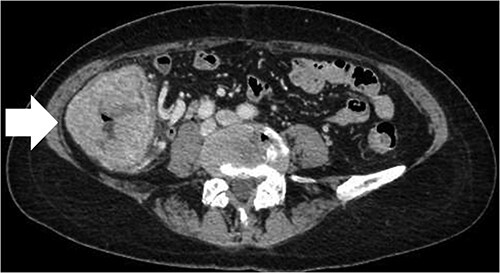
CT scan of the large tumor in the ascending colon (arrow).
